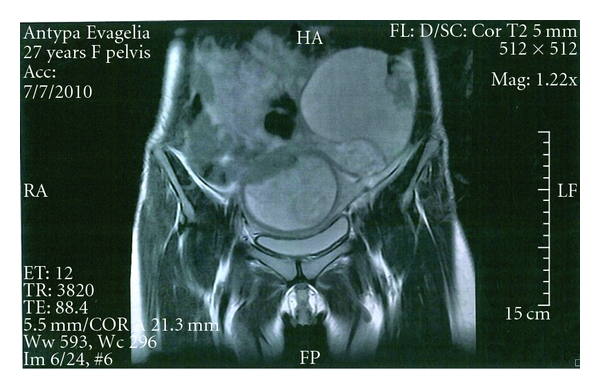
MRI of a mature cystic teratoma of the left ovary. A large cystic mass with component of high sigual intensity, consistent with fat.
